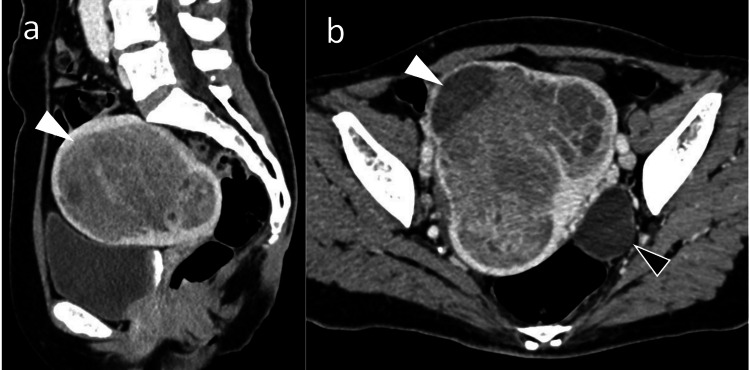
Pelvic computed tomography image Contrast-enhanced computed tomography images showing a heterogeneously enhanced mass (white arrowhead) within an enlarged uterus. There is also a left ovarian cyst that is not suspicious of malignancy (black arrowhead). (a) Sagittal sectional image; (b) axial sectional image.
